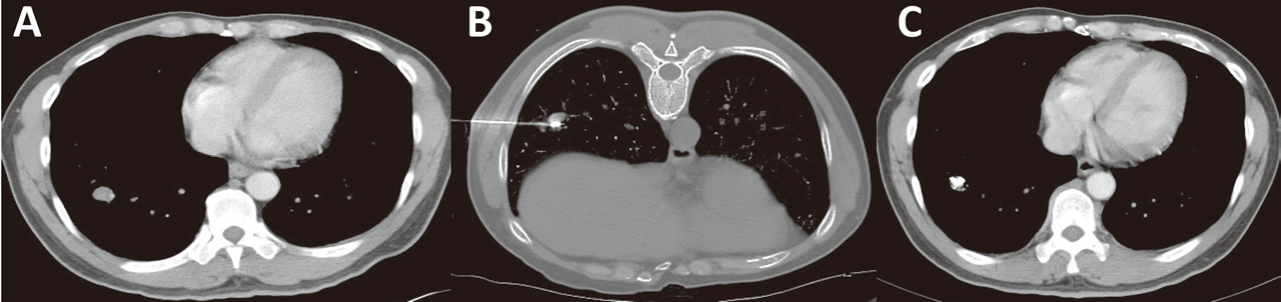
Minimally invasive treatment for HCC with pulmonary oligo-metastasis. (A) CT of an HCC patient showing newly developed right lung oligo-metastatic lesions. (B) Percutaneous particle implantation was performed. (C) A 6-month follow-up CT showed that the metastatic tumor had shrunk and exhibited no activity.
